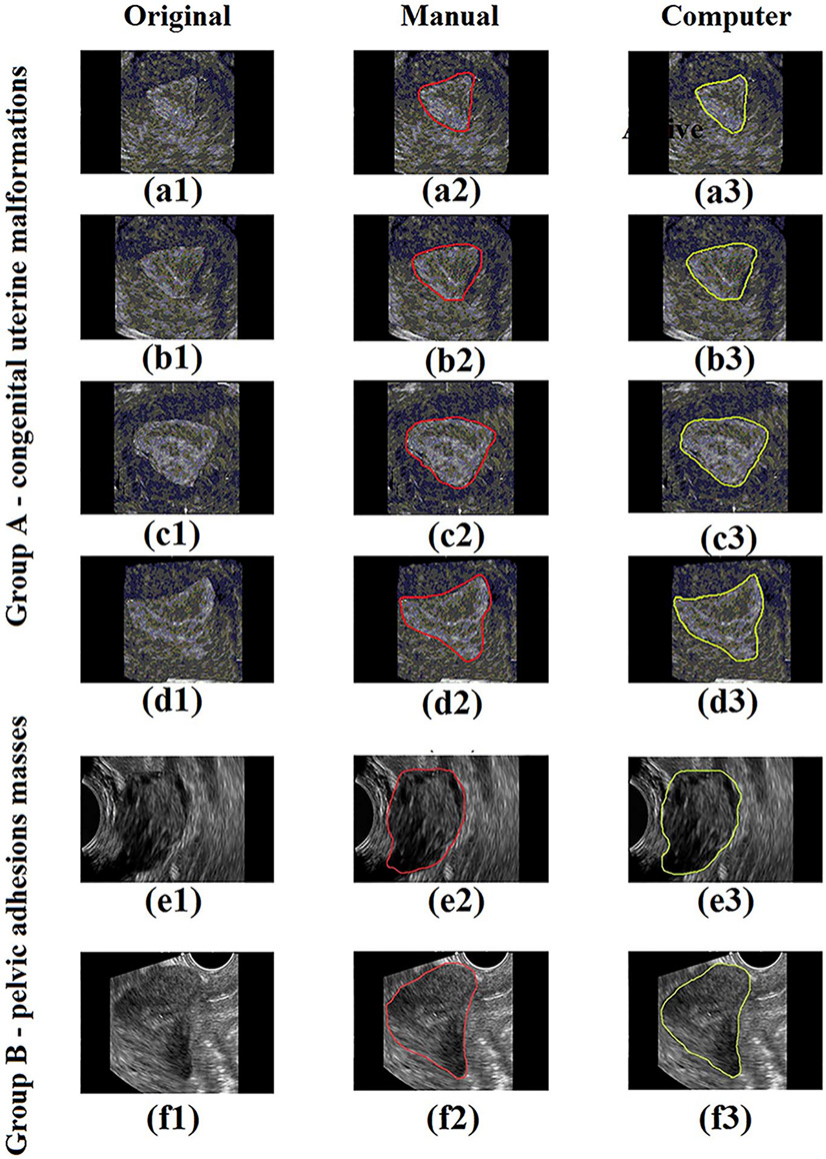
Gynecology ultrasound scans with segmentation of uterus by active contour model. (a1), (b1), (c1), (d1), (e1) and (f1) in the first column -are original images. (a2), (b2), (c2), (d2), (e2) and (f2) in the second column are images with uterus edge drawn by the doctor. (a3), (b3), (c3), (d3), (e3) and (f3) are images with uterus edge detected by our method. (a) to (d) represents Group A, and (e) to (f) represents Group B of our experiment.
